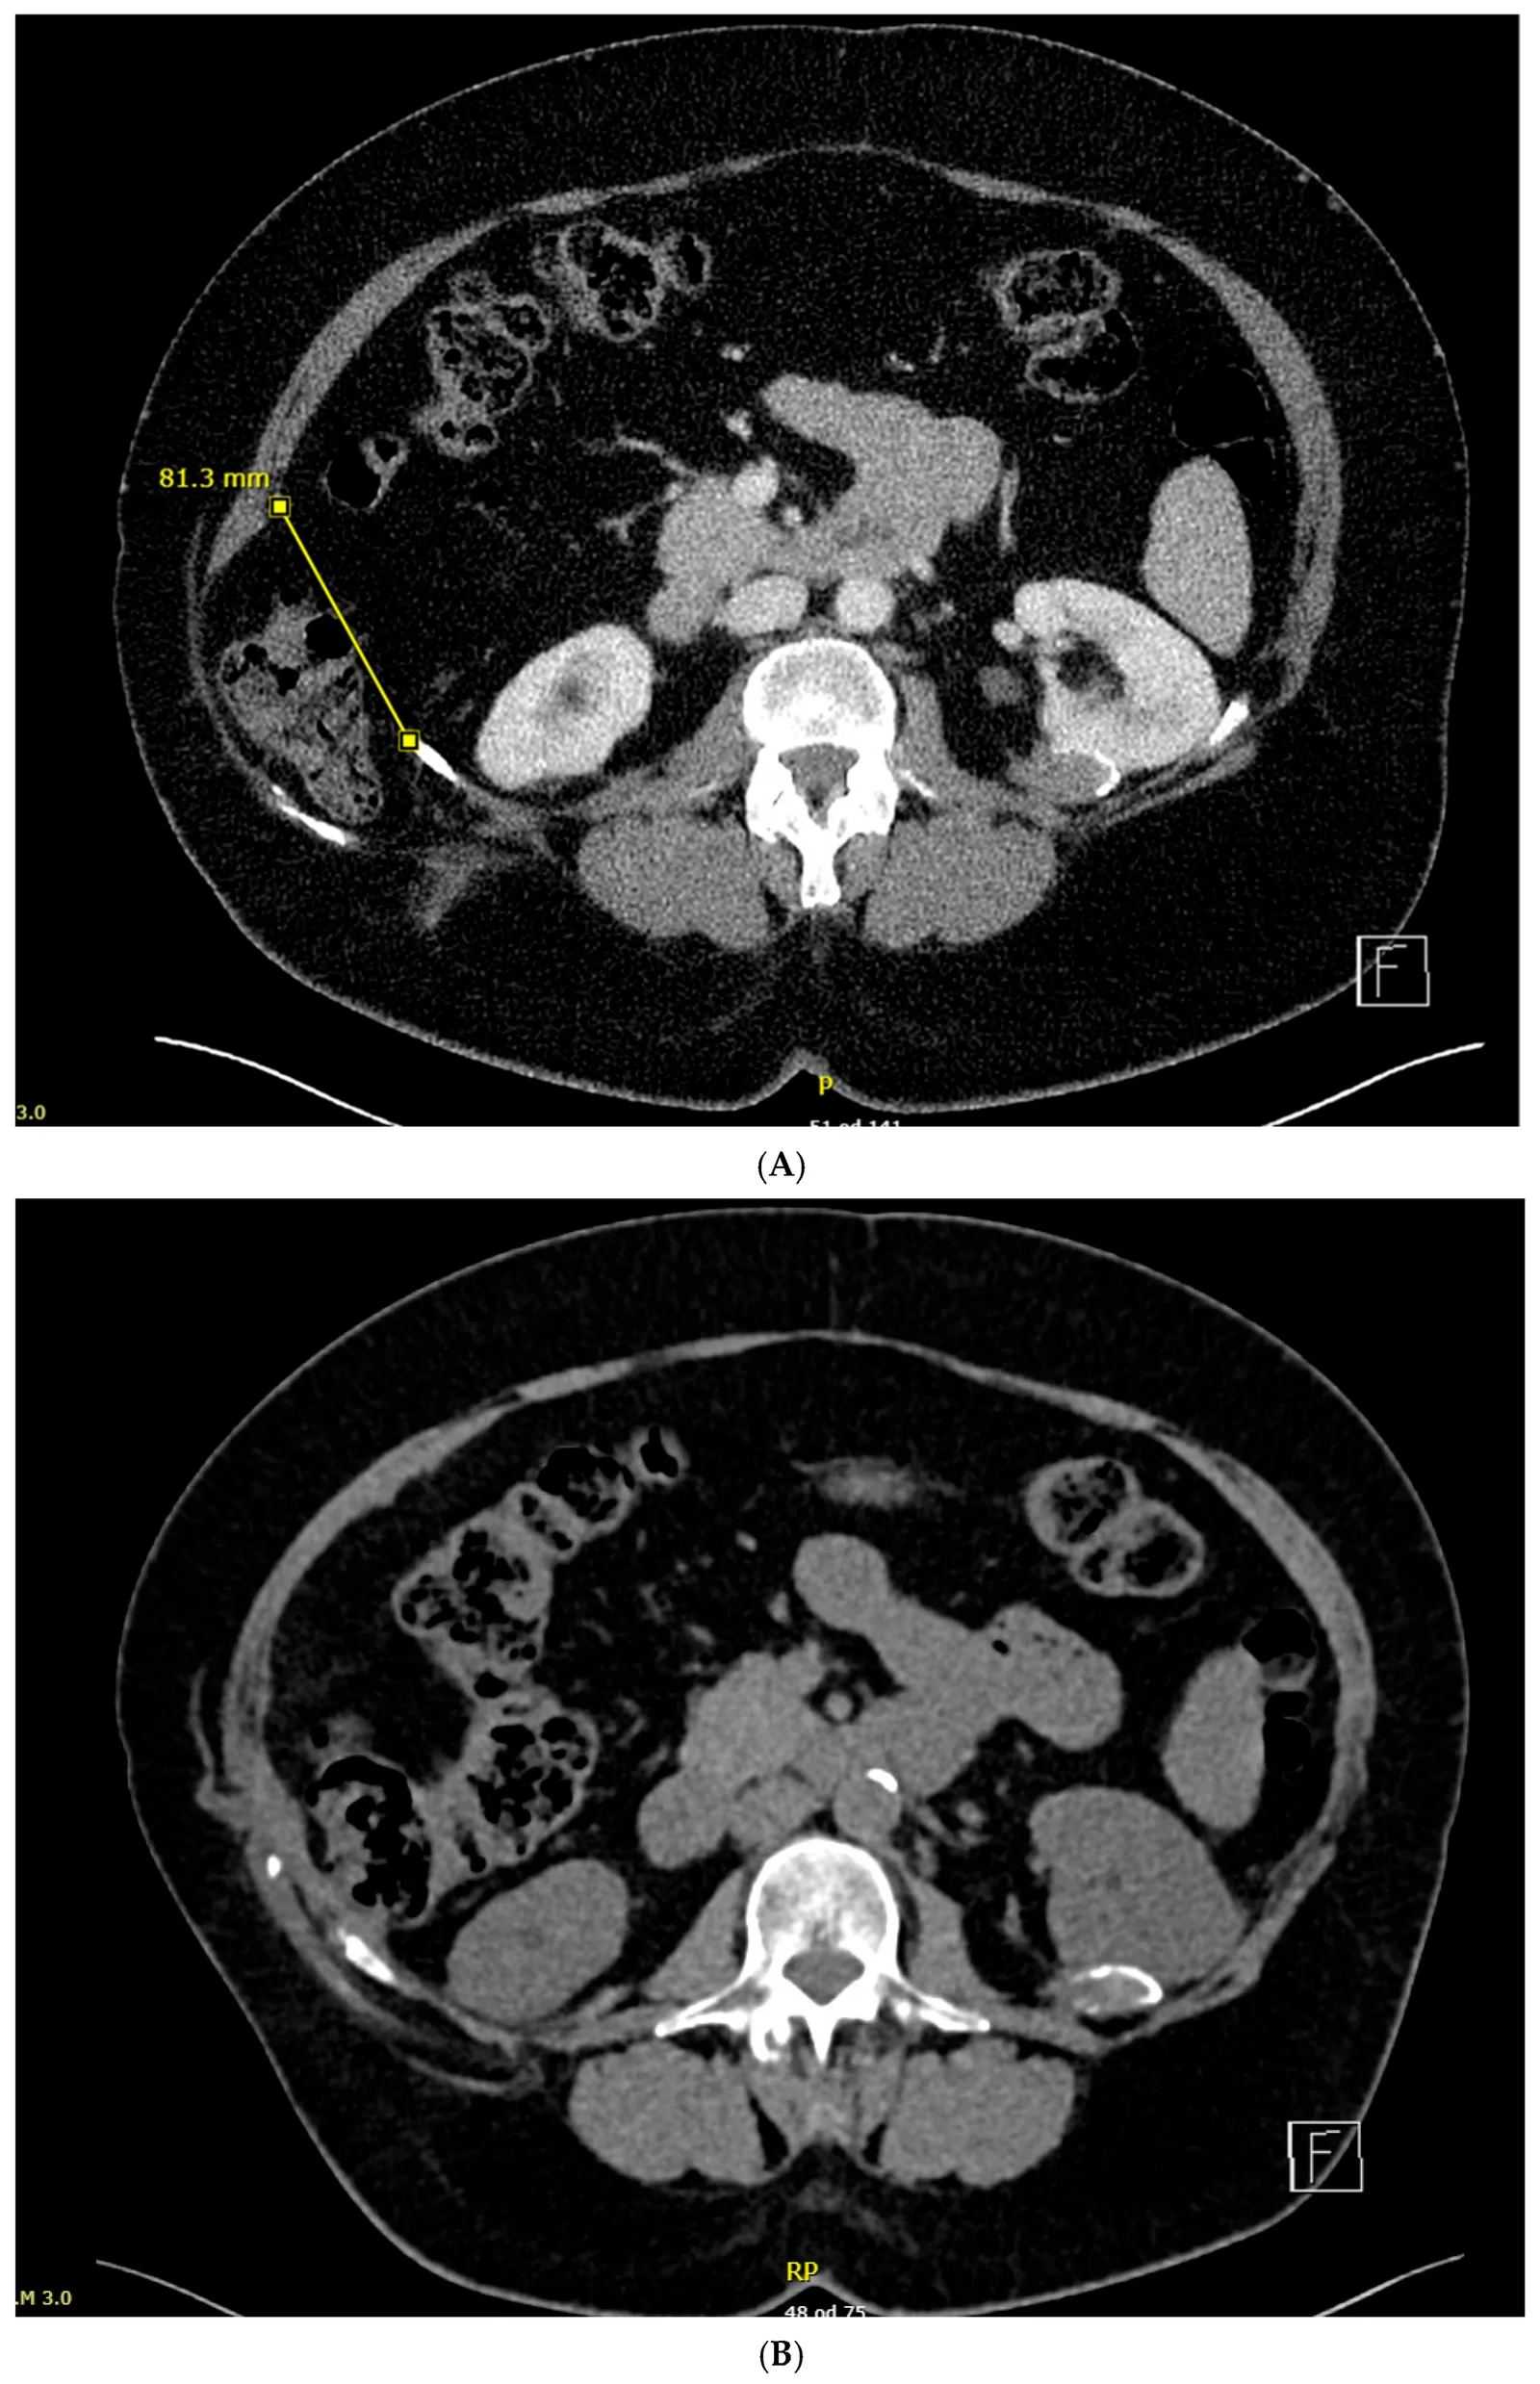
Representative computed tomography images of a recurrent lateral incisional hernia following lumbotomy for partial nephrectomy. (A) Preoperative axial CT demonstrating a recurrent lateral hernia after previous failed onlay hernioplasty. (B) Postoperative axial CT at 5 months following robotic preperitoneal repair performed with the patient in the lateral decubitus position, demonstrating successful abdominal wall reconstruction with mesh in the extraperitoneal position.
